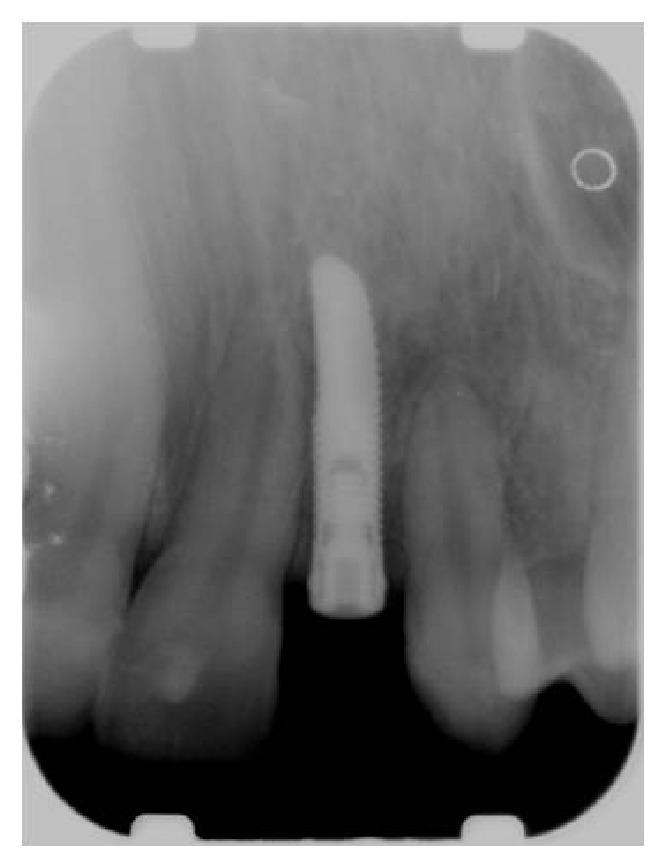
Radiographic control after implant placement in site to the right.
